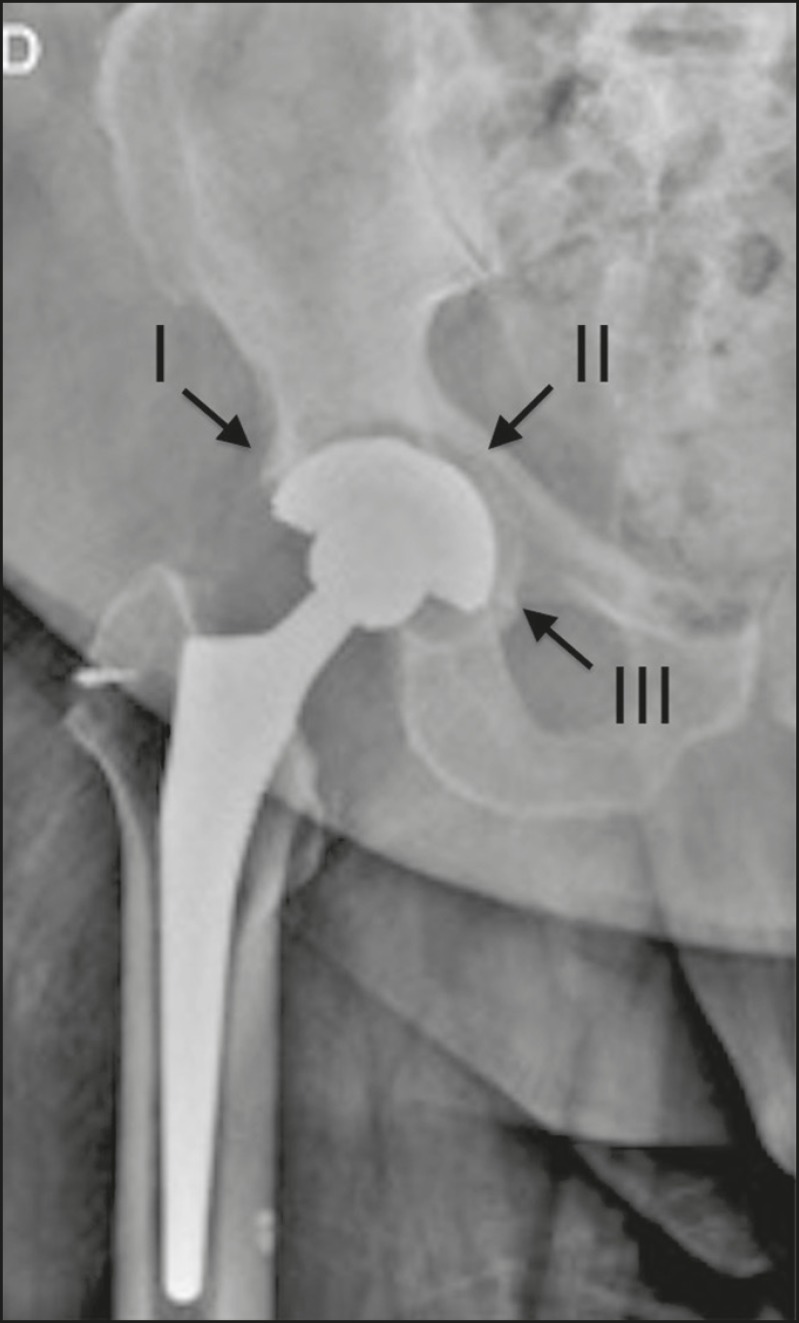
Anteroposterior X-ray of the right hip with osteolysis in zones I, II and III (arrows) of the right acetabular component.
